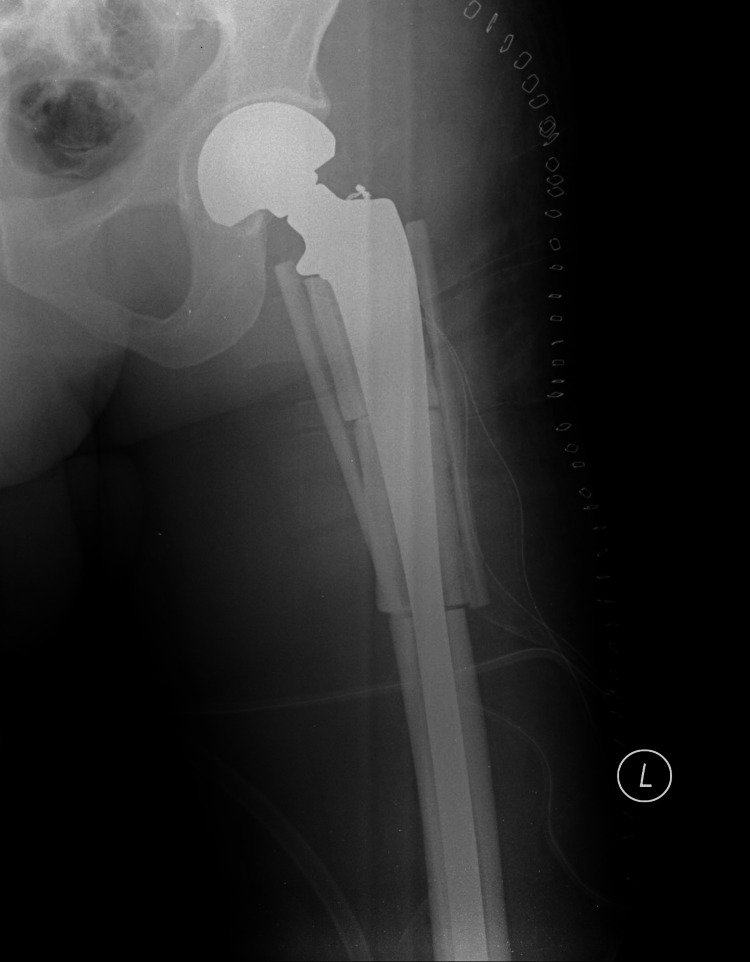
Day-one postoperative radiograph
